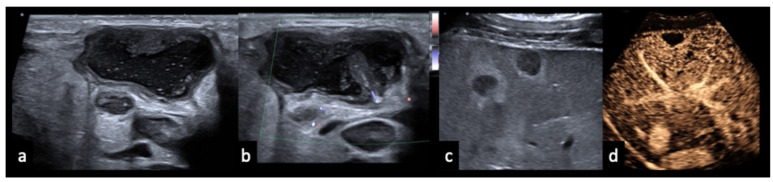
23-year-old male patient presenting with weakness, shortness of breath, and painful cervical lymph node swelling. (a) B-mode ultrasound shows a nearly anechoic cervical mass with floating internal echoes. (b) Color Doppler imaging demonstrates absence of intralesional vascularity. (c) Abdominal B-mode ultrasound reveals multiple hepatic lesions with varying morphology. (d) CEUS shows perilesional hyperperfusion in the arterial phase (30 s), while most of the mass appears non-enhancing, consistent with extensive necrosis. The final diagnosis of disseminated bartonellosis was established by serology. During medical therapy with doxycycline and rifampicin, the cervical abscess spontaneously perforated through the skin. Shortness of breath was attributed to acute myocarditis.
